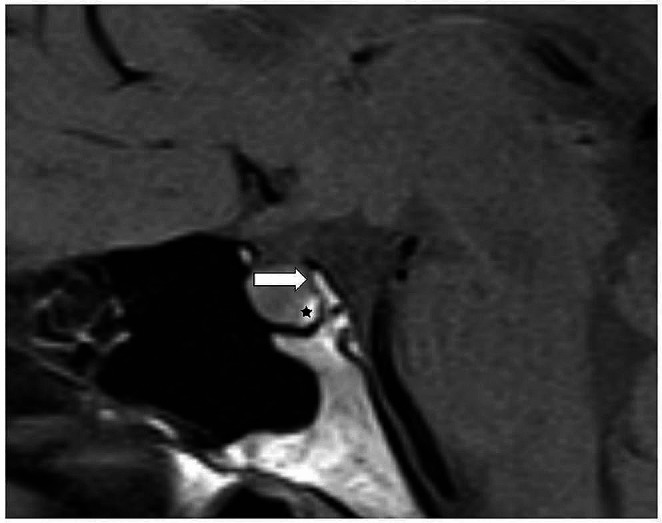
A T1-weighted sagittal image without contrast in a healthy person. The arrow marks the cortical layer of the clivus, which presents as a physiological border between bone marrow fat and the bright spot (*) of the posterior pituitary lobe
